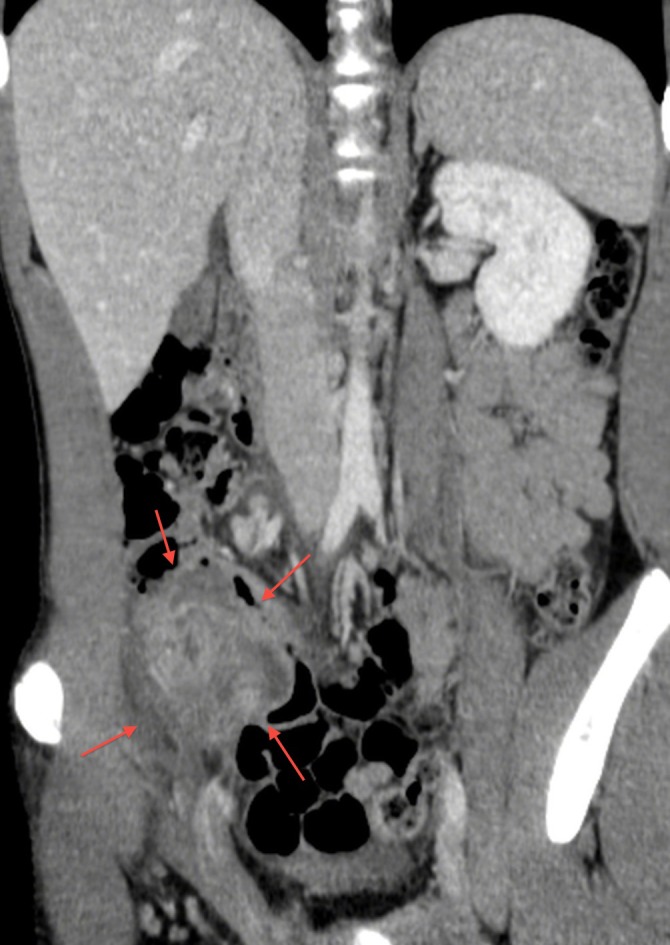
Figure 3:CT image of a mixed cystic and solid mass (6.5 cm x 6 cm x 4.2 cm) in the right lower quadrant with a calcified fecolith.
